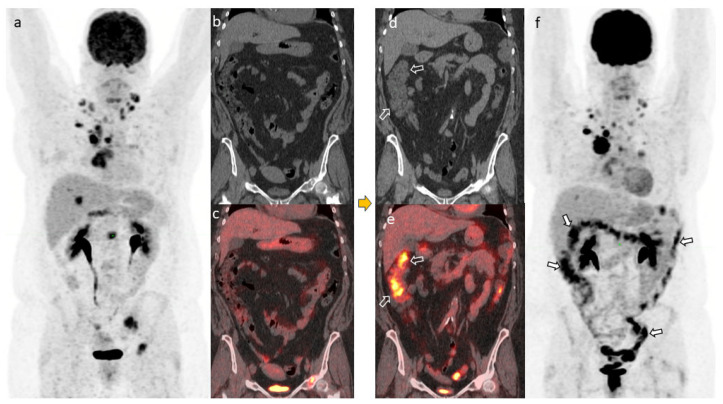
PET/CT scans in Case 1 prior to *Clostridioides difficile* diagnosis. The maximum intensity projection (MIP) image (a), coronal CT (b), and coronal PET/CT (c) showed no appreciable abnormal uptake in the colon. The PET/CT scans 1 month after treatment of diagnosed *C. difficile*. The coronal CT (d), coronal PET/CT (e), and MIP image (f) showed diffuse FDG uptake in the colon but without corresponding wall thickening on CT (arrows in (d–f)). This type of uptake was deemed nonspecific stimulation/inflammation from immunotherapy but was not severe enough to meet the diagnosis of colitis.
